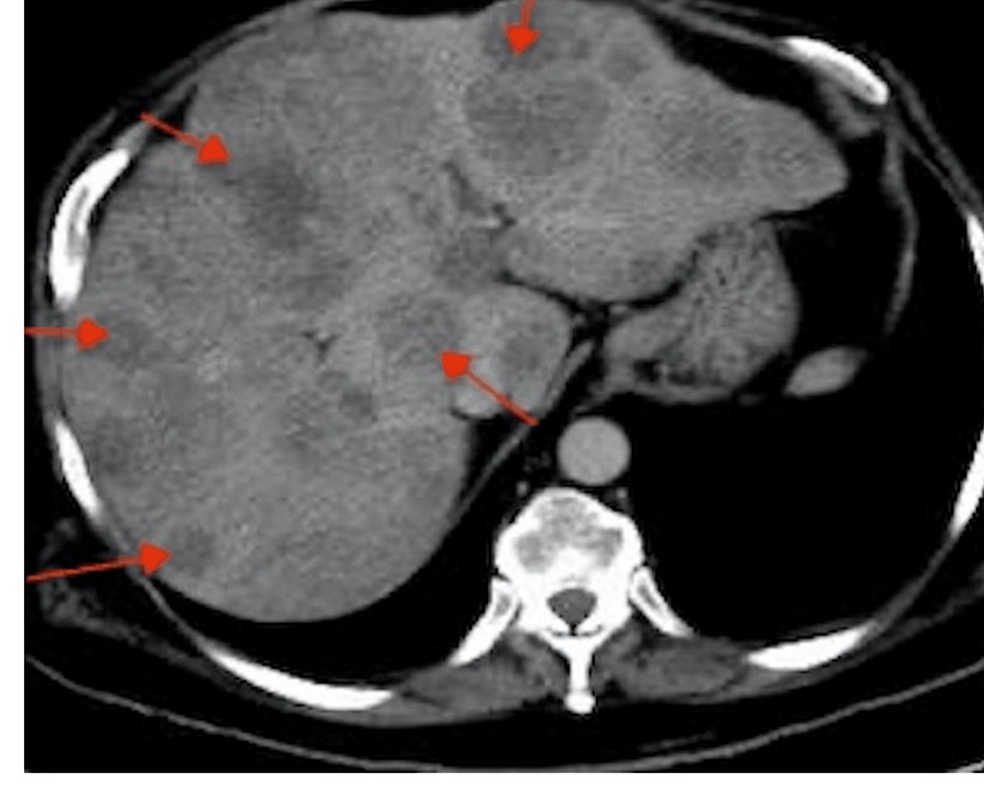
Abdominal computed tomography shows multiple hypoattenuating lesions (red arrows) in the liver, indicating metastatic disease.
